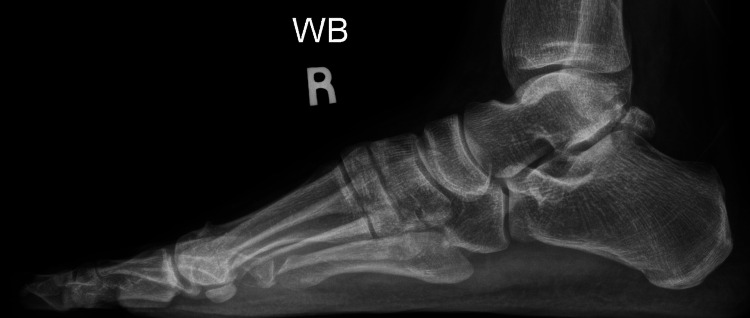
Pre-operative weightbearing lateral foot radiograph
